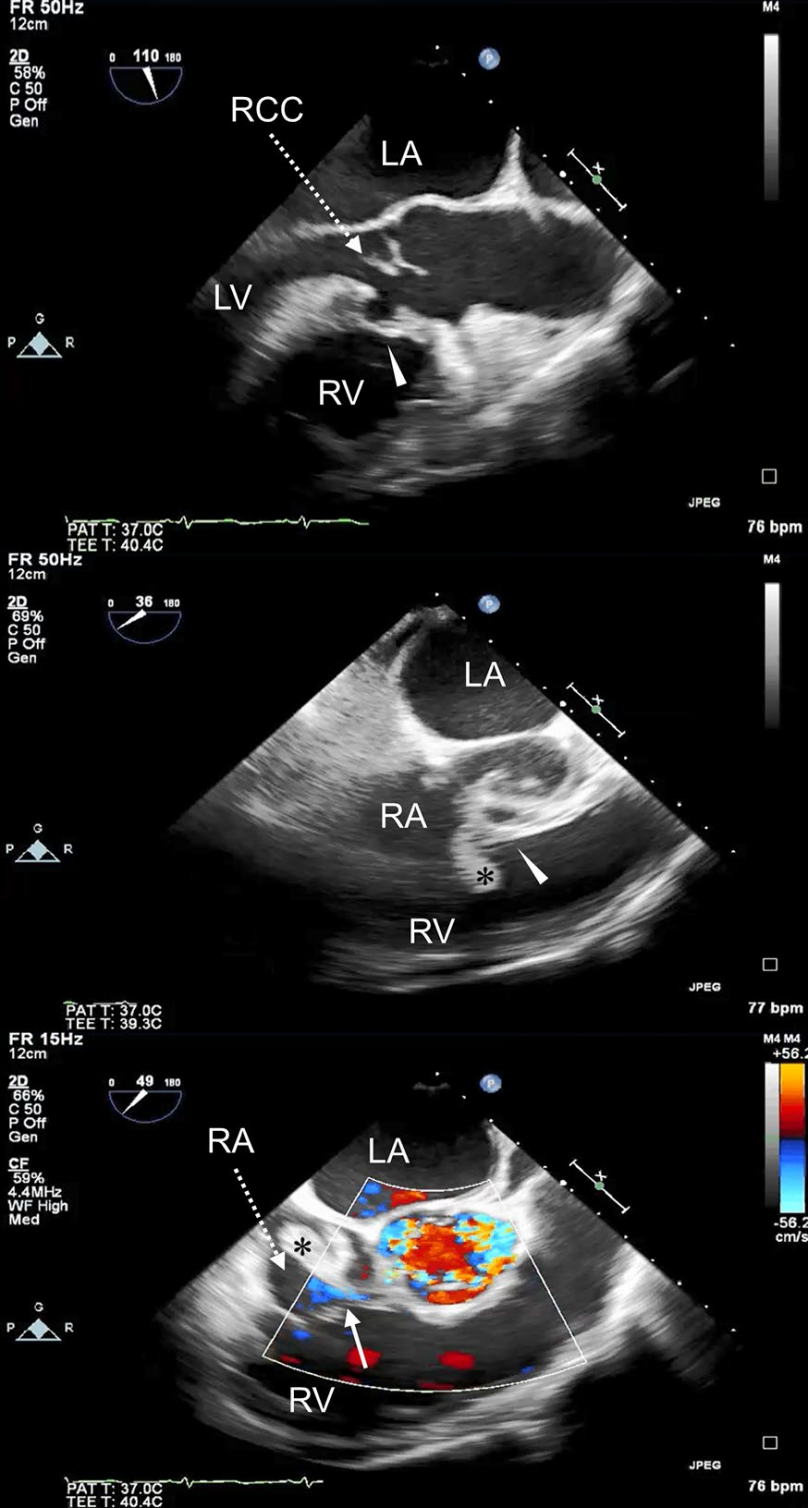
Preoperative TEE demonstrating detachment of the right coronary cusp of the aortic valve from the annulus and the cavity immediately below the right coronary cusp (white arrowhead). The vegetation was detected on the atrioventricular septum (black asterisk). Color Doppler imaging demonstrating systolic blood flows from the LV into the RA through the cavity (white arrow). LA: left atrium; LV: left ventricle; RA: right atrium; RV: right ventricle; RCC: right coronary cusp
